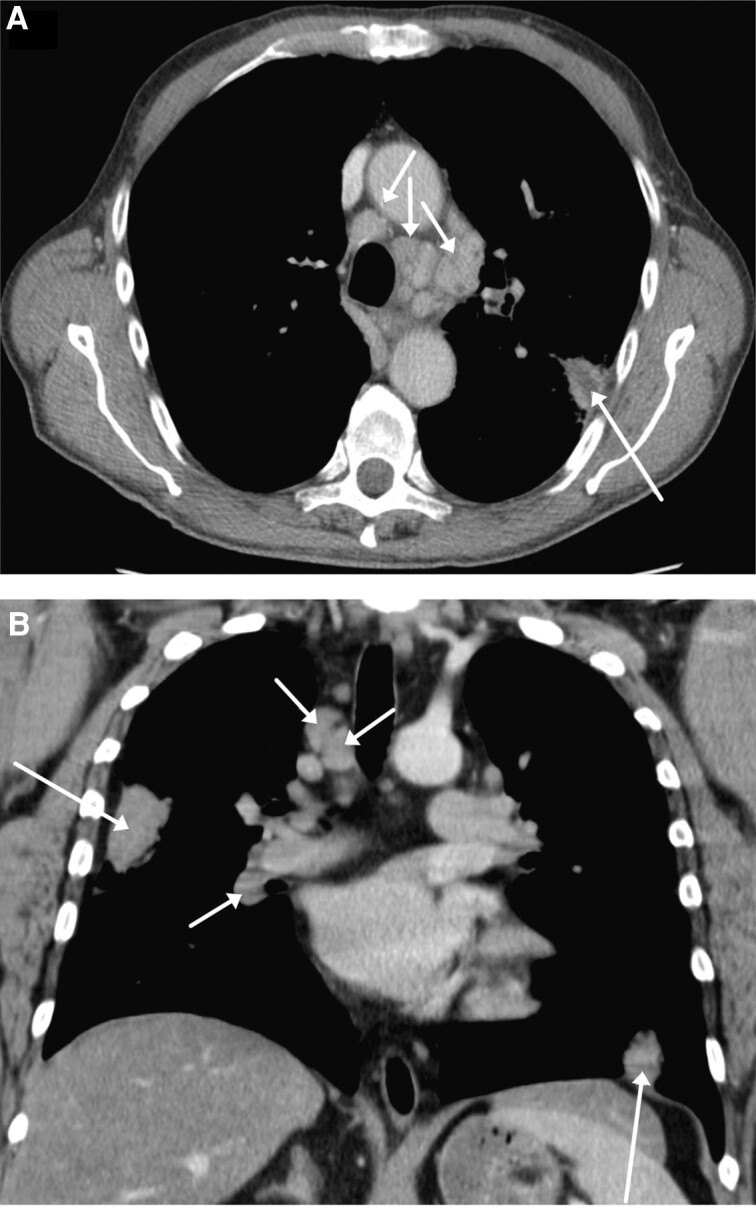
A,* Axial computed tomography section of the thorax of a 68-year-old male case-patient 10 days after disease onset. A 2.5-cm subpleural consolidation with central necrosis is indicated by the long arrow. Mediastinal reactive lymph nodes are indicated by short arrows. *B,* Coronal computed tomography section of the thorax of a 48-year-old male case-patient 7 days after disease onset. Over the previous 24 hours, he developed a dry cough. Bilateral subpleural consolidations are indicated by long arrows. Moderately enlarged paratracheal lymph nodes are indicated by short arrows. Image credit: Region Jämtland-Härjedalen.
